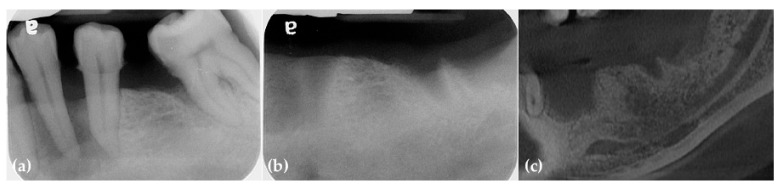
(a) Severe bone loss caused by advanced periodontal disease is observed around the first and second premolars; (b) The preoperative radiograph reveals severe vertical bone loss due to advanced periodontal disease following extraction; (c) The preoperative panoramic CBCT scan demonstrates significant vertical bone defects and extraction socket defects. The inverted letter a indicates the orientation mark of the radiograph.
